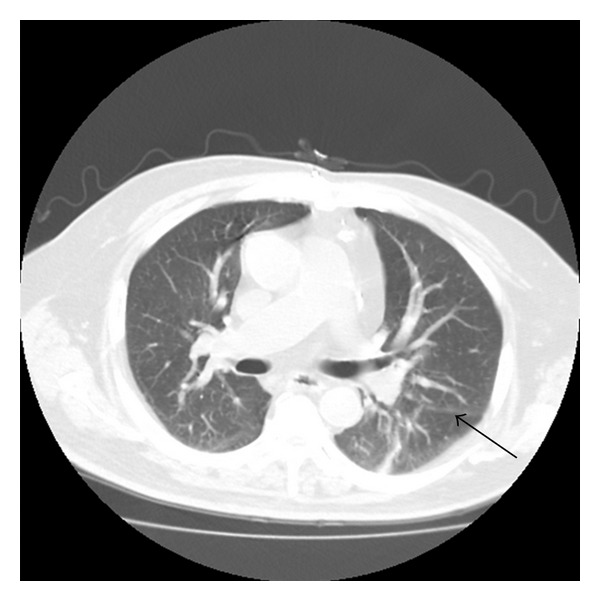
Chest computed tomogram with intravenous contrast three months after admission with resolved ground glass infiltrates revealing the initial atelectasis of the left lower lobe present two months prior to admission (arrow).
